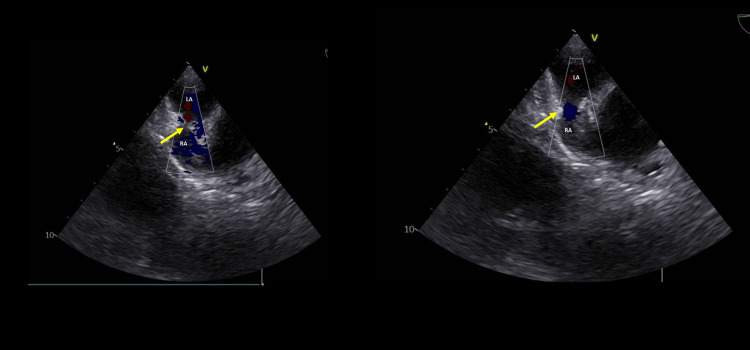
Two-dimensional Doppler echocardiogram. RA: right atrium. LA: left atrium. The yellow arrow indicates blood flow (color Doppler) through the patent foramen ovale.
